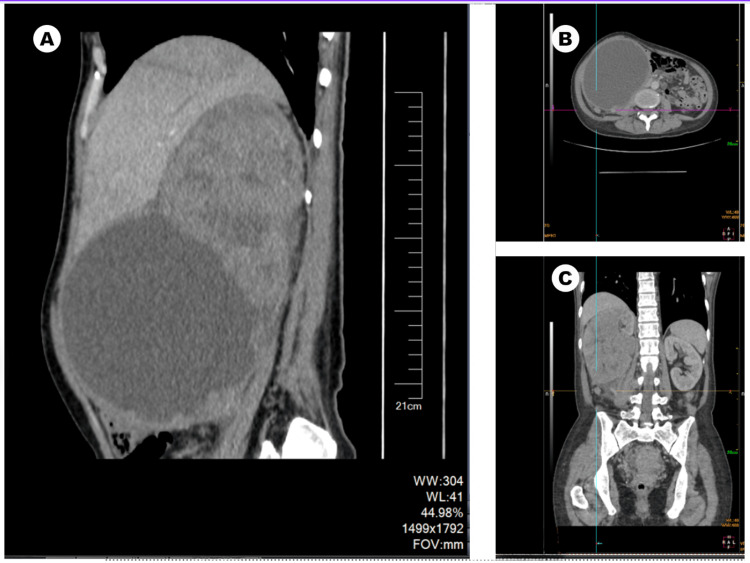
Abdominal CT scan with contrast of the patient. (A) Sagittal plane of the abdominal CT scan showing the mass; (B) transverse plane; (C) coronal plane.
